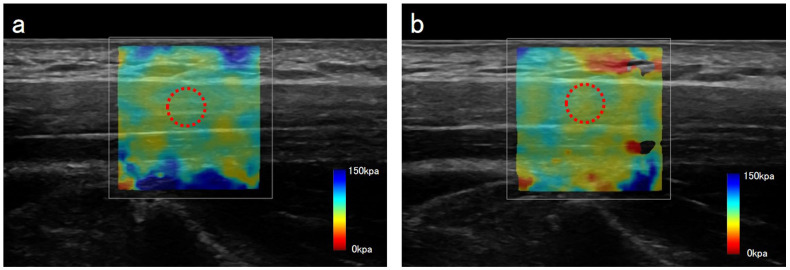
Shear wave elastography images of a healthy adult participant at baseline (a) and after upper trapezius ultrasound therapy (b). The color map is set such that blue indicates high trapezius stiffness and red indicates low trapezius stiffness. The region of interest was adjusted to muscle thickness, and a central circular Q-box was placed.
